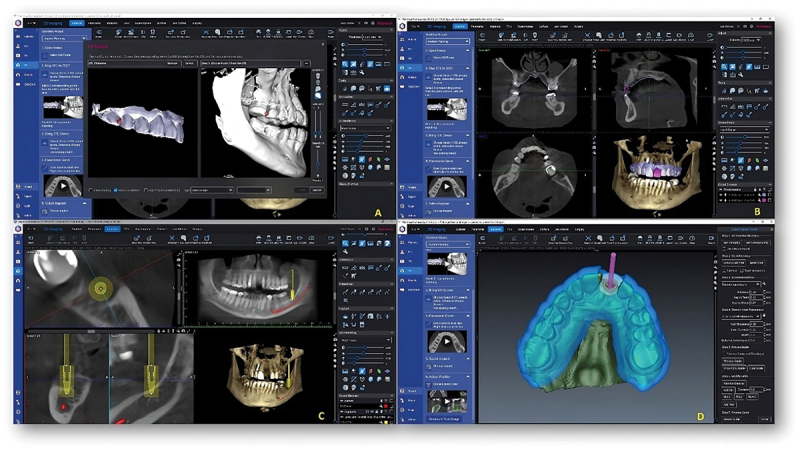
Showing the basic steps in virtual implant planning procedure. A-Mapping the intraoral scan with CBCT. B-Mapping of virtual crown with CBCT. C- position the implant using implant centric view. D- preparation of the virtual surgical guide.
